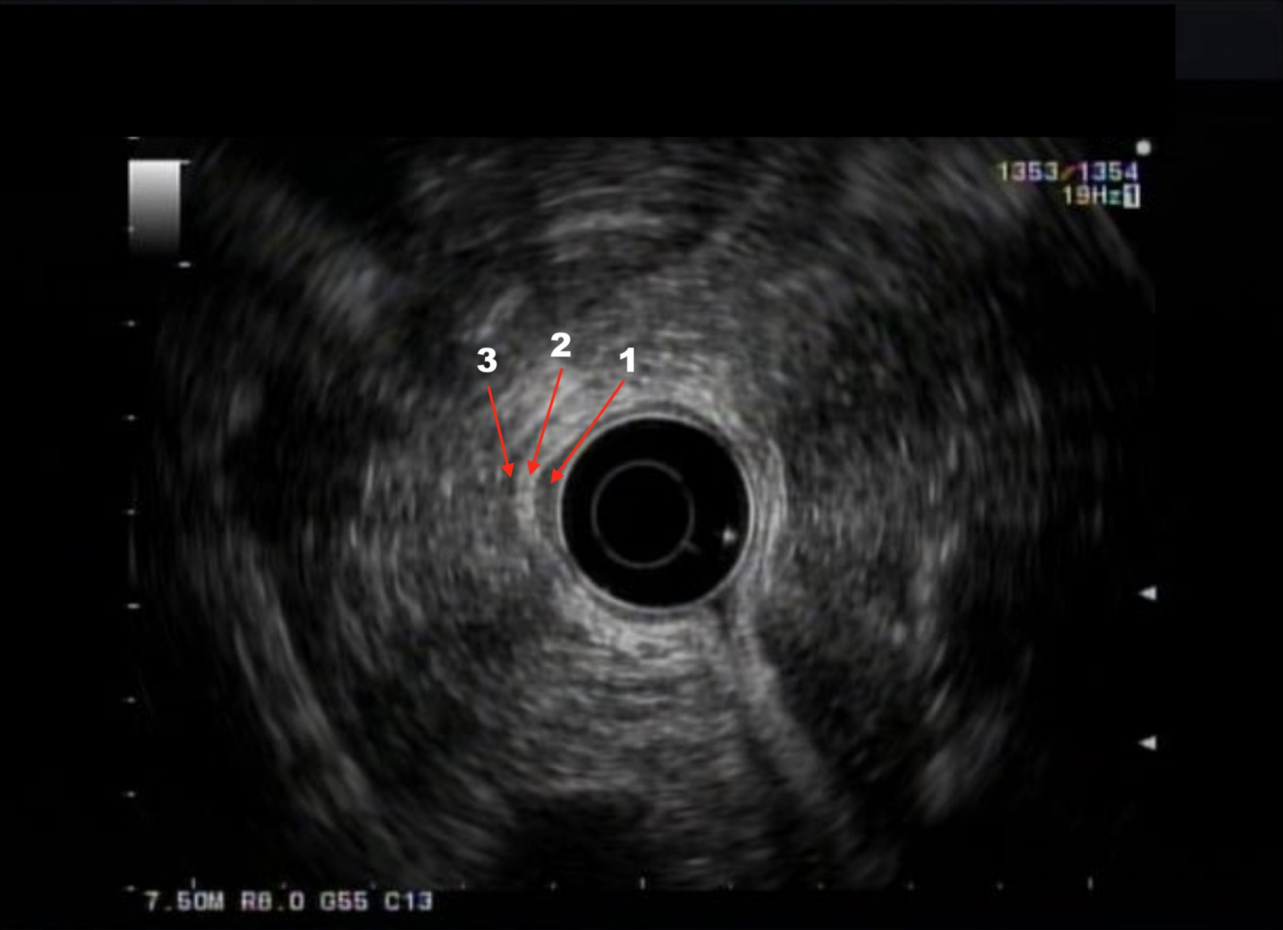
Endoscopic ultrasound (EUS) imaging EUS showing isolated mucosal nodule (arrow number 1) with no deep invasion of the submucosa (arrow number 2) or muscularis propria (arrow number3)
